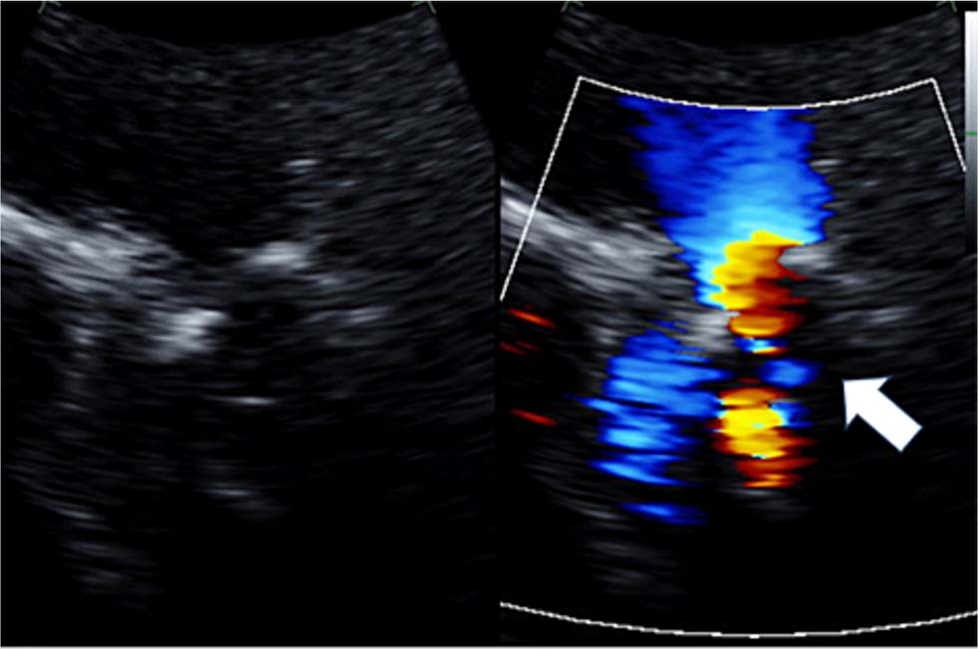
Preoperative transthoracic echocardiography: moderate pulmonary regurgitation. The regurgitant jet is indicated by the white arrow.
